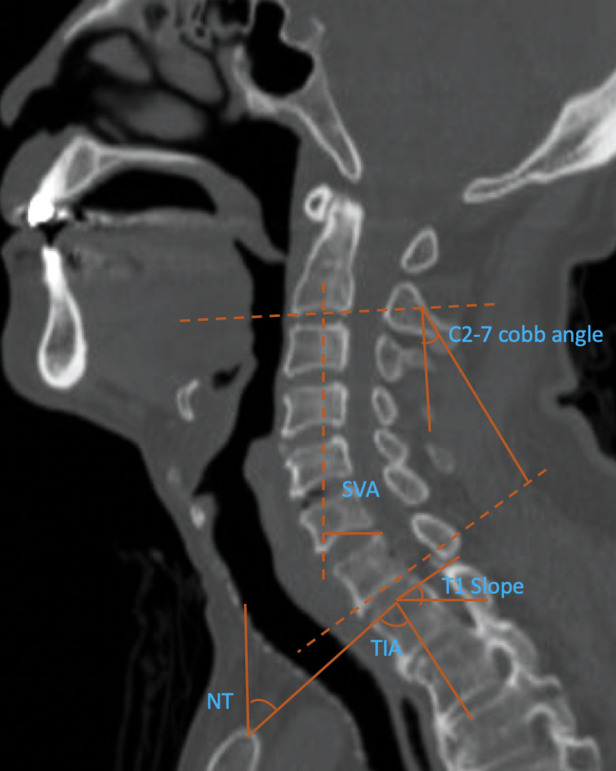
Radiographic evaluation of T1 slope, NT (neck tilt), and TIA (thoracic inlet angle), C2–7 Cobb angle, and C2–7 SVA (sagittal vertical axis).
